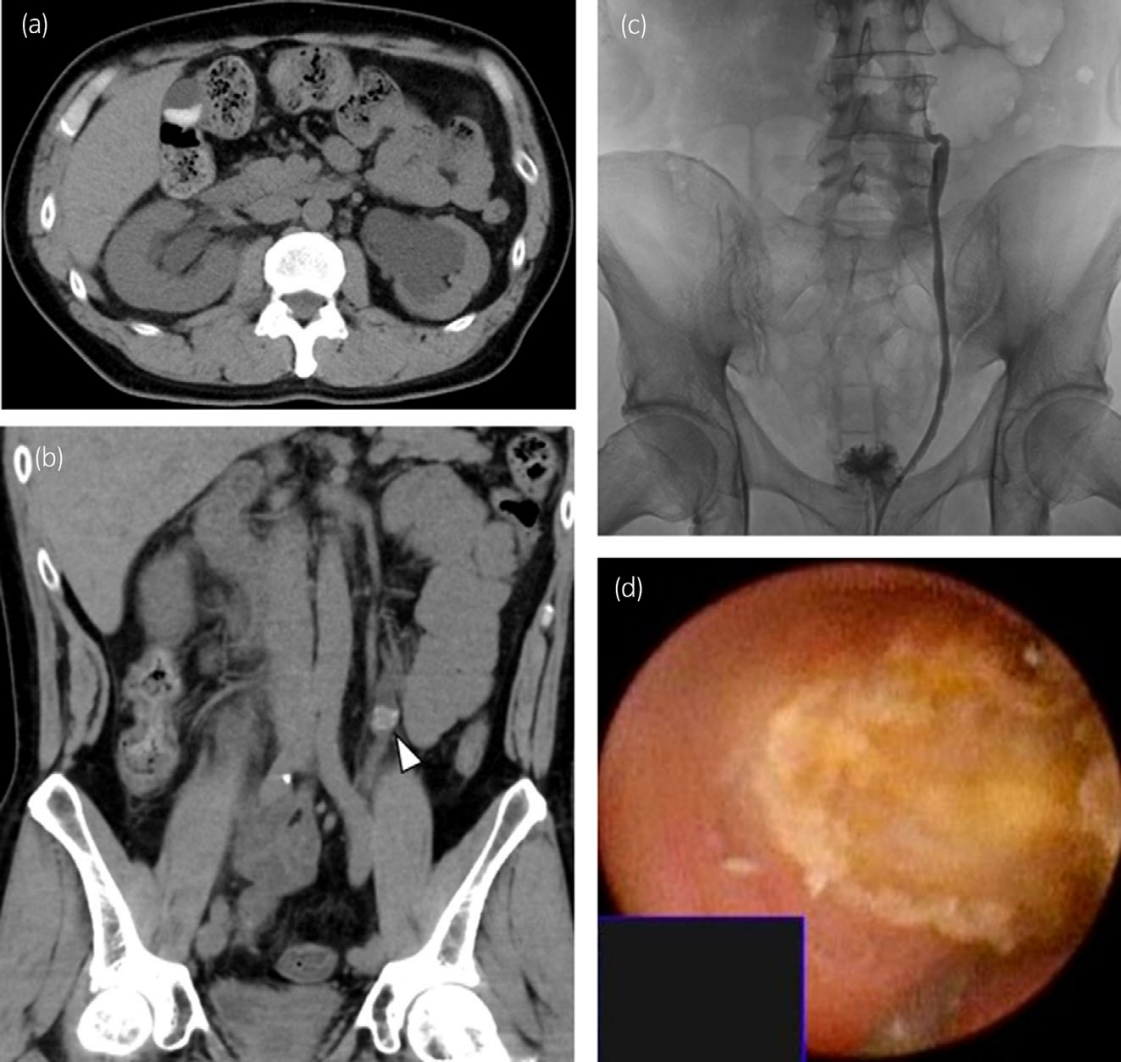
Plain CT images of the bilateral hydronephrosis with left‐sided predominance and thinning of the cortex of the left kidney (a) and the mass (arrowhead) in the left upper ureter (b). X‐ray image during retrograde ureterography. The contrast medium was stopped at the site of the mass (c). Endoscopic image of the left ureteral stone during lithotripsy (d).
